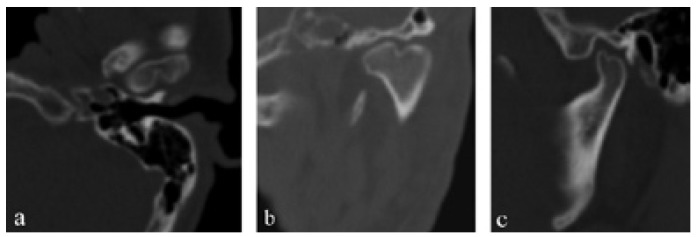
Axial a) coronal b) and sagittal c) CT scans (Patient-6) revealed left-sided BMC in a bilateral case.
